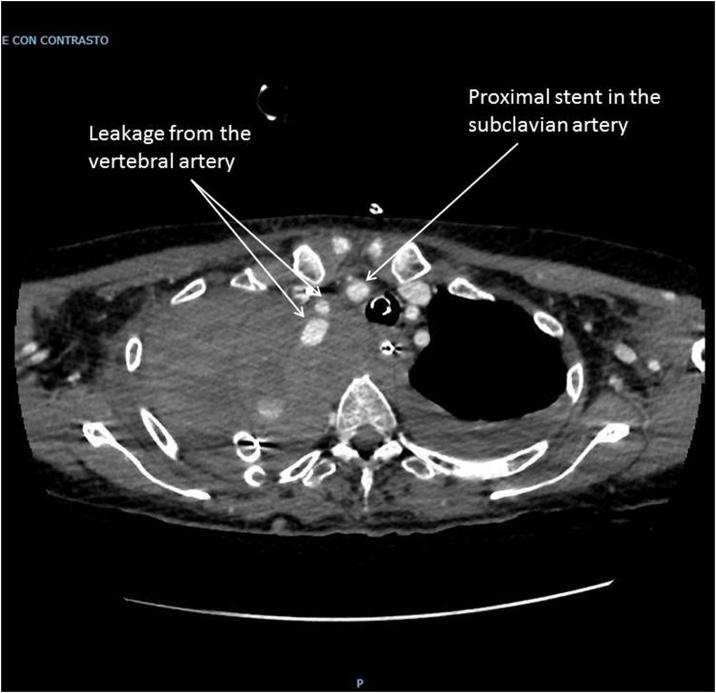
CT scan showing leakage from the stent, due to reperfusion of the vertebral artery a few hours after the angiographic procedure.
